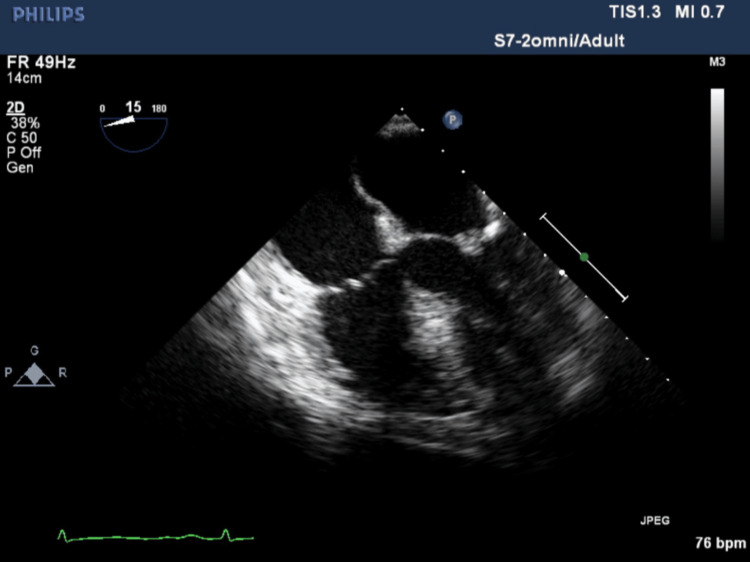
Mitral valve vegetation as small mobile mass on the thickened mitral valve in on transesophageal echocardiogram
